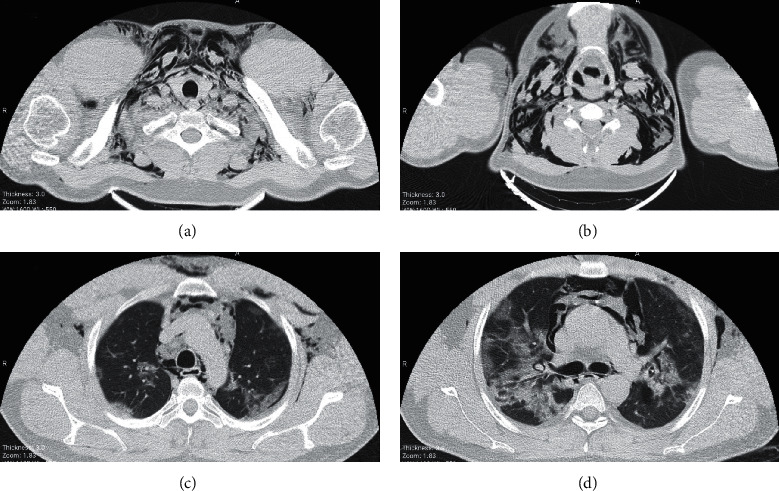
Axial images (a–d) of pulmonary computed tomography in the parenchymal window from the thoracic inlet and upper chest depict extensive pneumomediastinum, pneumothorax, and soft tissue emphysema. Note the peripheral patches of consolidation/ground-glass density.
